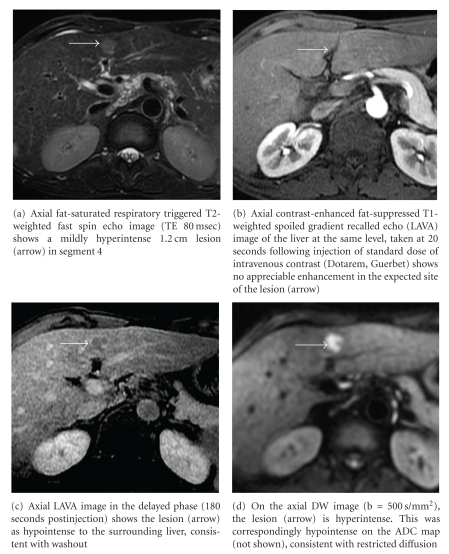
HIV positive patient with chronic HBV infection without known liver cirrhosis. By the AASLD and APASL guidelines, this lesion would require further evaluation. CT done prior to the MRI also failed to demonstrate arterial hypervascularity. Note, however, that the lesion showed suspicious features on T2-weighted and DW imaging. The lesion was biopsied percutaneously under ultrasound guidance and showed to represent a well-differentiated HCC.
